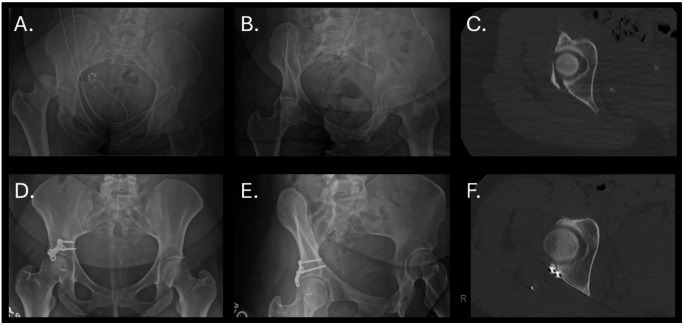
Case example. A Anteroposterior radiograph of an isolated posterior wall fracture dislocation. B Obturator oblique radiograph of the posterior wall fracture following closed reduction. C Axial computed tomography demonstrating cranial and peripheral nature of posterior wall fracture. D Post-operative anteroposterior radiograph. E Post-operative obturator oblique radiograph. F Post-operative axial computed tomography demonstrating near anatomic wall reduction and peripheral plate positioning
